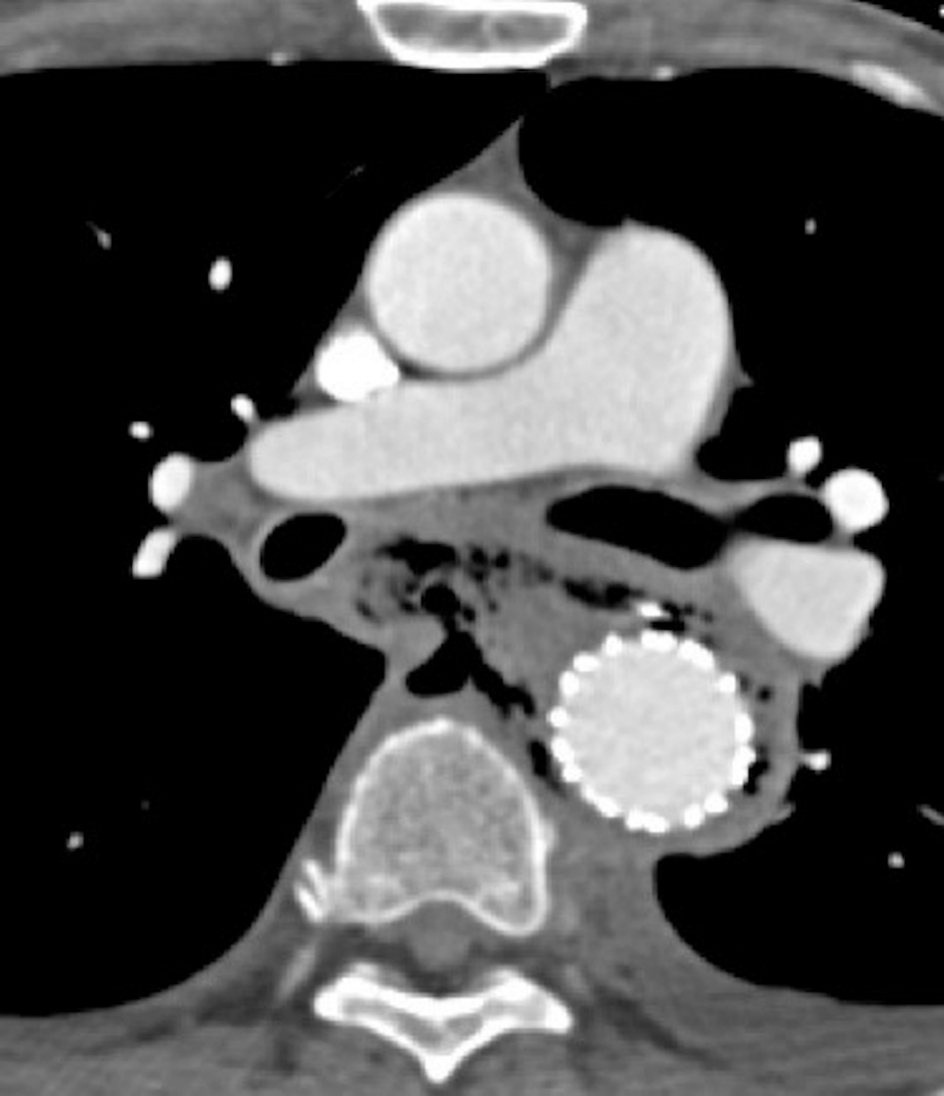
Axial CECT also showing large pneumomediastinum within the middle and posterior mediastinum surrounding the endovascular graft, with discontinuity of the posterolateral esophageal wall. CECT, contrast-enhanced CT.
